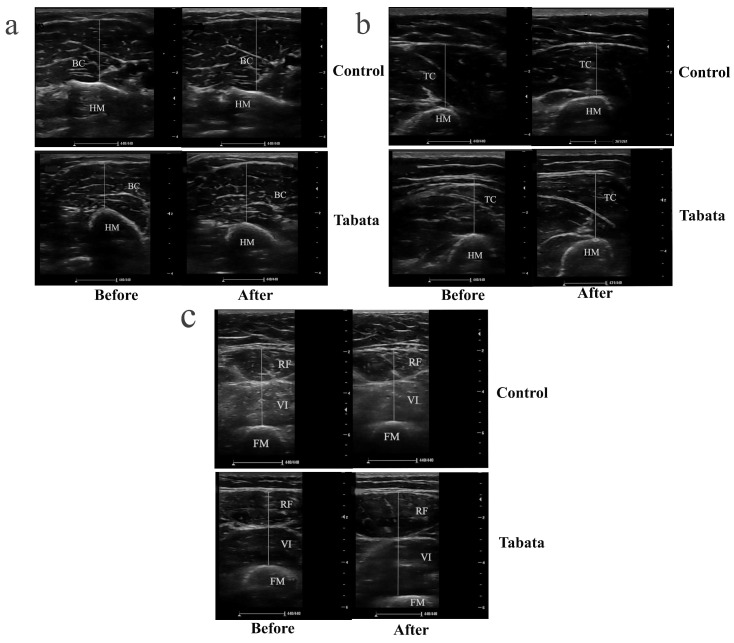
Ultrasound images demonstrating muscle thickness of participants in control and Tabata groups before and after 12-week intervention. (a) Transverse scan of biceps muscle (BB), with the hyperechoic, curvilinear structure in the lower part of the image representing the humerus (HM). (b) Transverse scan of triceps muscle (TC), where the hyperechoic, curvilinear structure in the lower part of the image corresponds to the HM. (c) Transverse scan of quadriceps muscle, comprising rectus femoris (RF) and vastus intermedius (VI); the hyperechoic, curvilinear structure in the lower part of the image represents the femur (FM). Solid straight lines indicate the method used to measure the thickness of each muscle.
